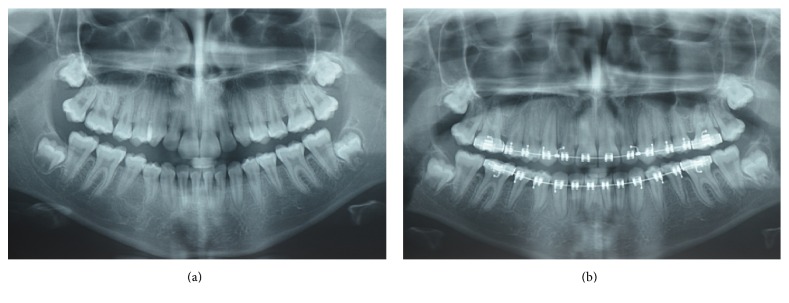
Panoramic radiographs of the patient aged (a) 12 years and 5 months and (b) 13 years and 7 months with no evidence of further supernumerary teeth.
